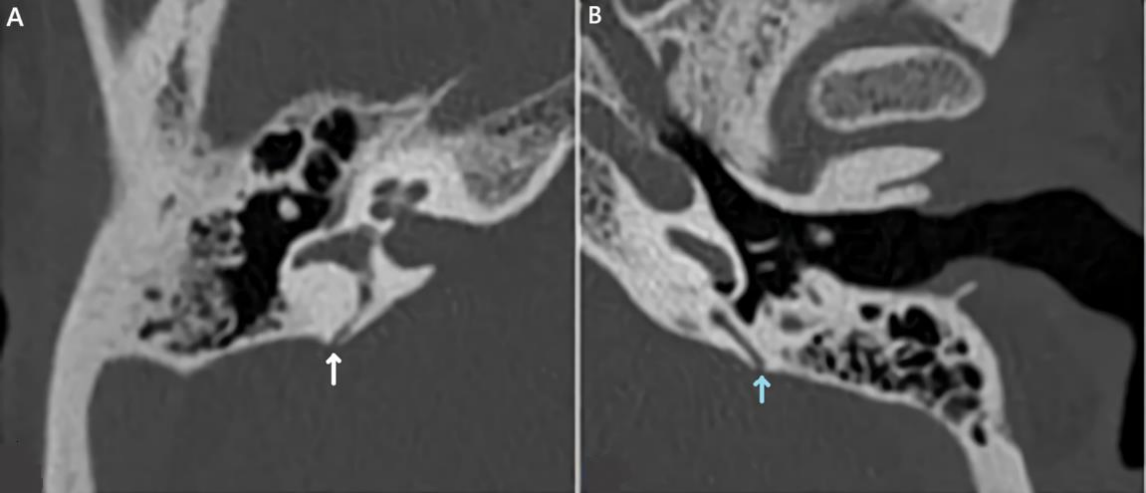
axial view of the patient´s temporal bone CT scan showing dehiscence of the right posterior semicircular canal (A); and (B) of the left posterior semicircular canal
